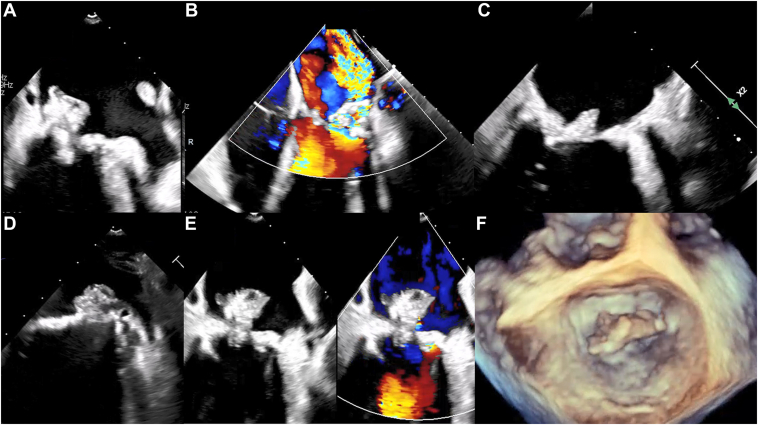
Example of infective endocarditis in patients with mitral annular calcification (MAC) using transesophageal echocardiography. (A) Large heterogeneous vegetation on the medial side of the mitral annulus. (B) Valvular perforation, with severe mitral regurgitation on the lateral side of the mitral annulus. (C) Heterogeneous hypodense vegetation on MAC. (D) Succulent heterogeneous vegetation on MAC. (E) Color comparison of the mitral valve showing a large heterogeneous vegetation overlying the calcified annulus. (F) Large vegetation visualized on 3-dimensional transesophageal echocardiography.
